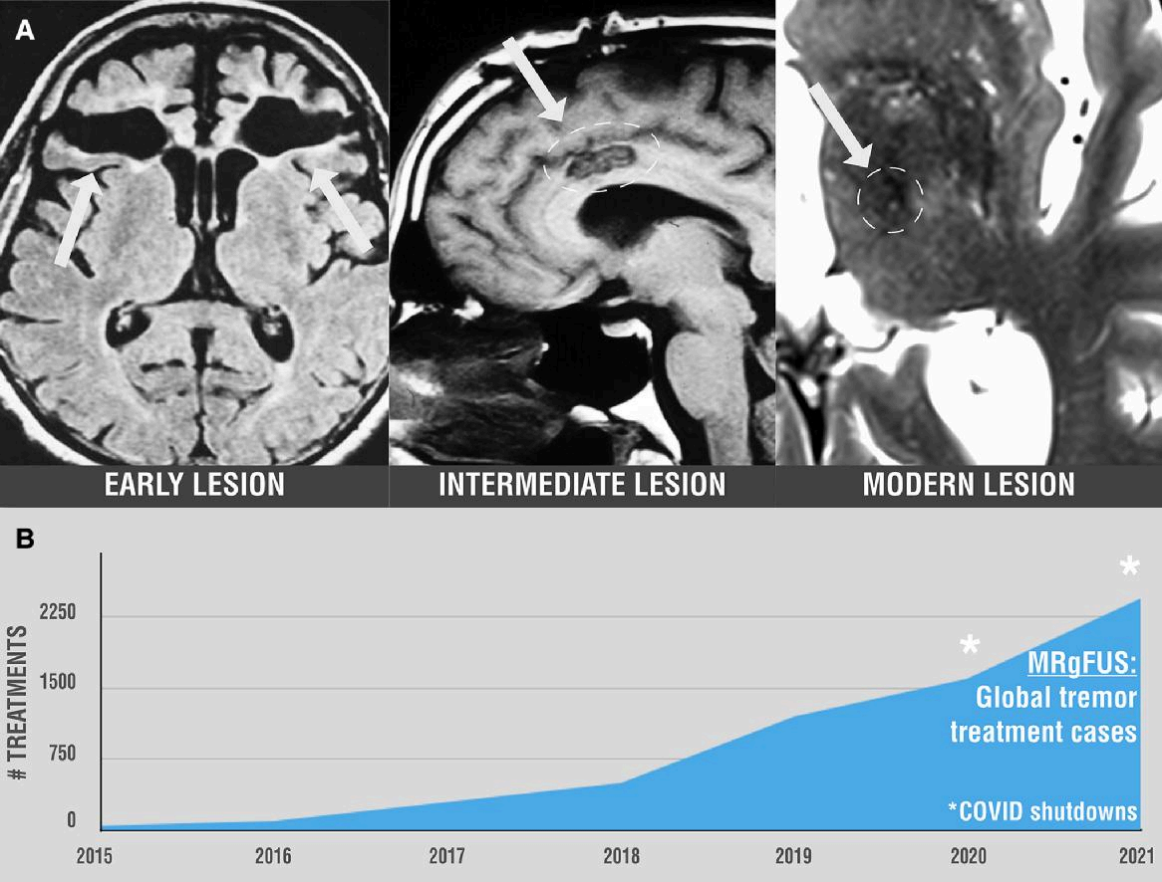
Evolution of therapeutic lesions in functional neurosurgery. (A) Examples of lesions from different time points in history. Early ablative lesions such those resulting from prefrontal leucotomy (*left*) were large and spanned multiple brain regions.^62^ After introduction of stereotactic frames around 1947, lesions became more precise, such as those generated by stereotactic cingulotomy (*middle*). Current lesions generated by modern ablative surgery are small, precise, and even barely visible on MRI just 3 months after the procedure, such as lesions created by magnetic resonance-guided focused ultrasound (MRgFUS) (*right*). Frontal lobotomy: Image distributed under a CC-BY-3.0 license, courtesy to Frank Gaillard. Cingulotomy: Image distributed under a CC Attribution-Share Alike 4.0 license, courtesy of operativeneurosurgery.com. (B) The number of MRgFUS procedures for tremor have increased rapidly over the past few years. Data provided by InSightec for the commercial neuro-exablate system.
